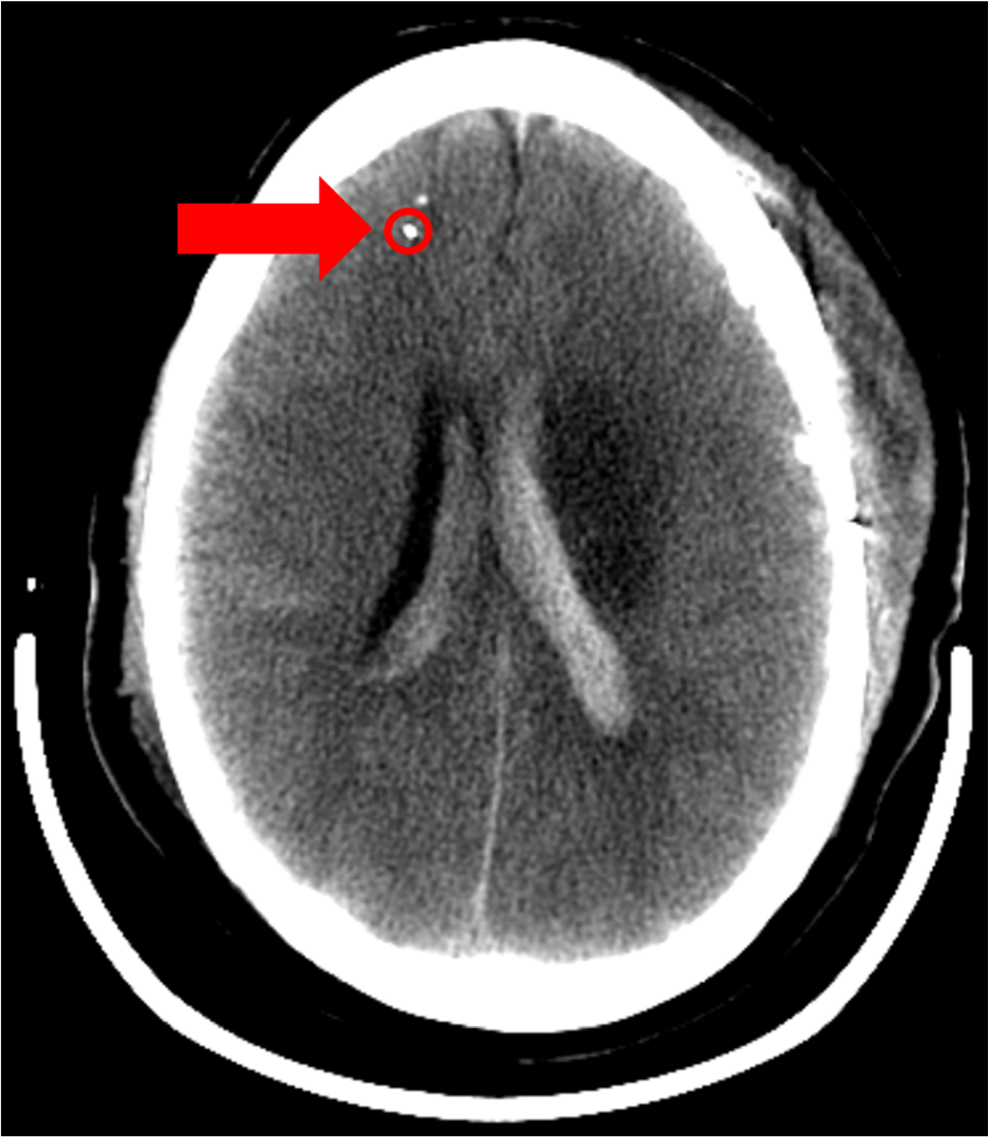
Probe placement with a CT scan from the patient where the red circle marks the optical probe positioned next to a microdialysis catheter
